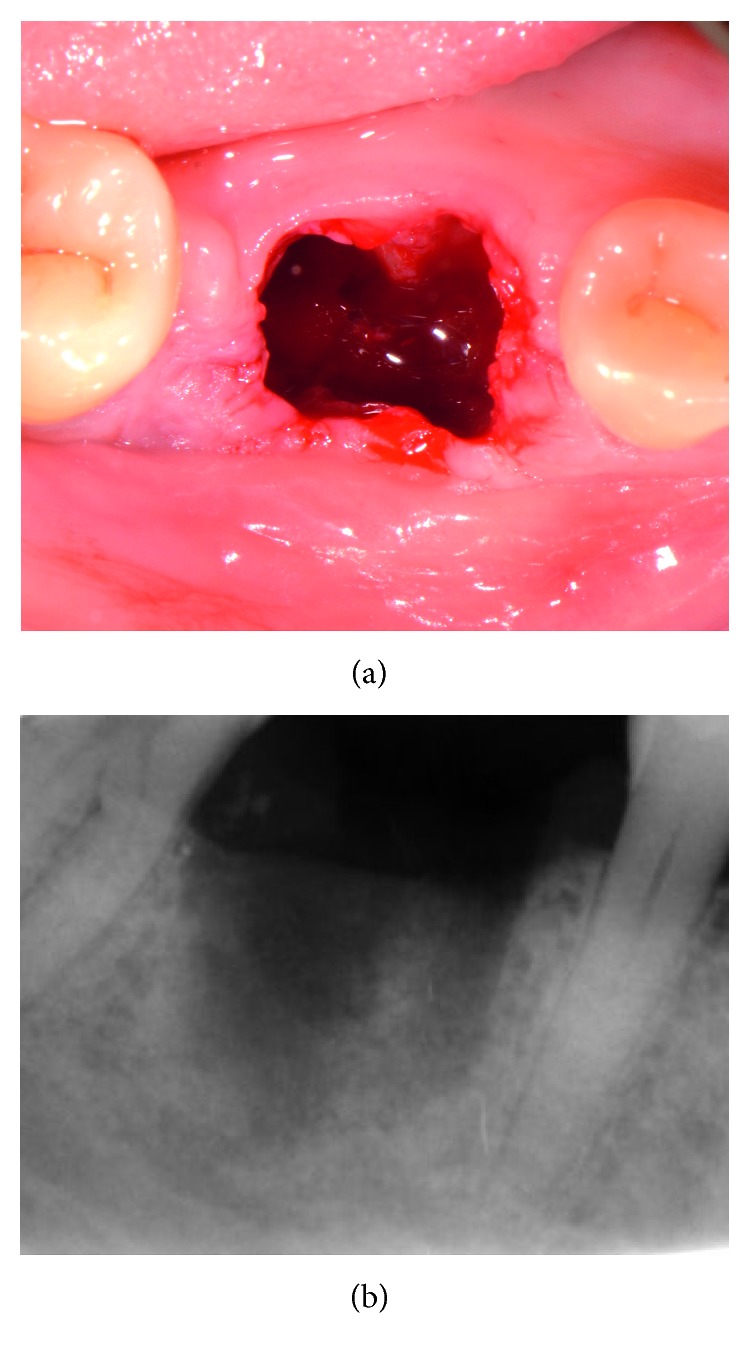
Clinical view and periapical X-ray of the site immediately after the “atraumatic” flapless extraction. The septal bone is completely missing, and the buccal bone was defective. Note the shortage of buccal keratinized soft tissue.
